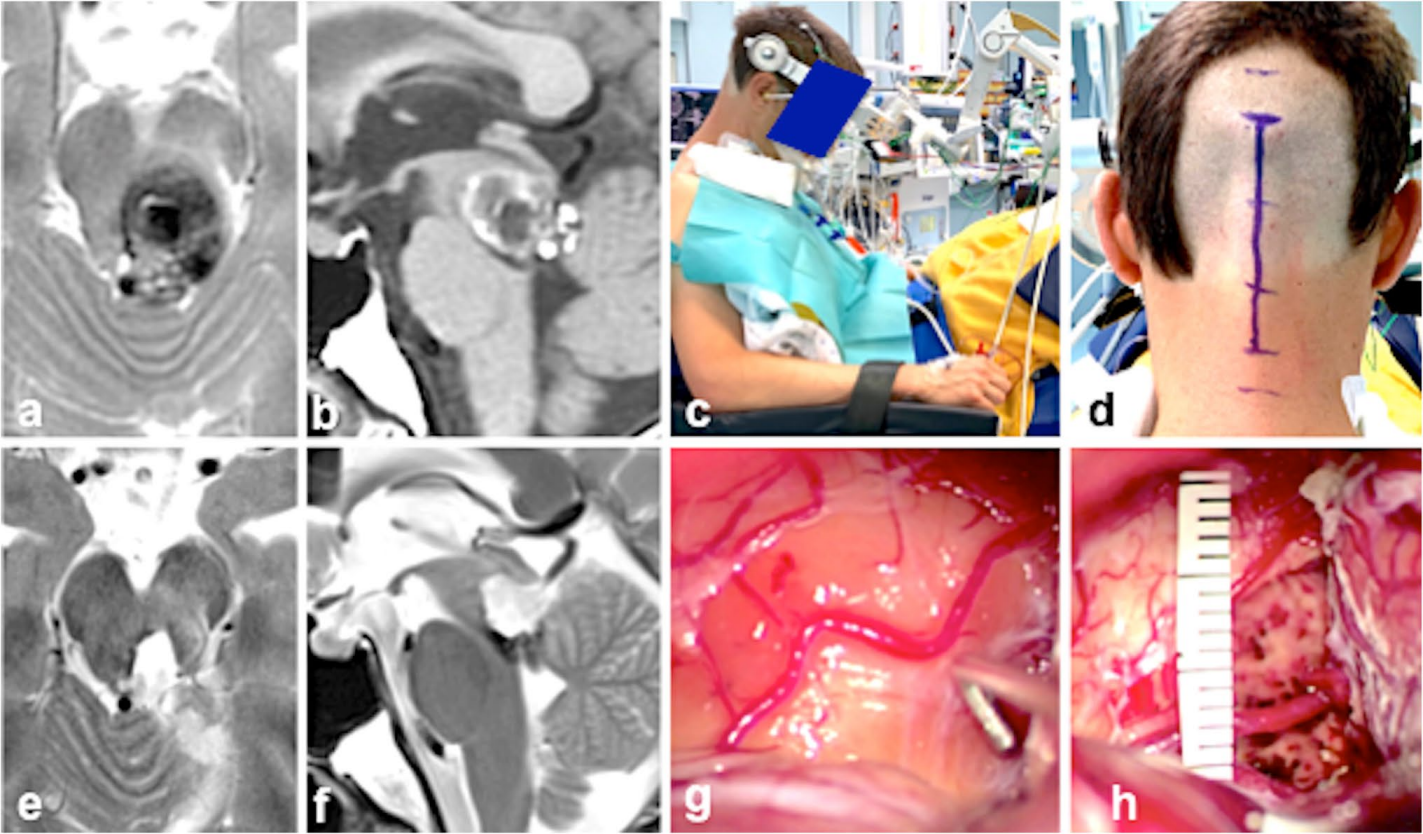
Over 5 years, this 35-year-old male suffered from recurrent headache and diplopia. Three months before surgery, the eye movement disorder worsened, and additional progressive gait instability was noted; the symptoms were caused by midbrain hemorrhage that gradually increased to a remarkable size as seen on preoperative axial (a) and sagittal (b) MRI. The patient underwent surgery in the semi-sitting position with the head flexed (c); a longitudinal midline occipital/suboccipital incision was marked on the skin (d). Postoperative axial (e) and sagittal (f) MRI confirmed total MCM resection. At surgery, the surface of the right midbrain tectum was prominent, but the underlying MCM was still covered by a thin layer of parenchyma (g). Total lesionectomy was also confirmed in surgery; the pial midbrain aperture measured 12 mm (h)
